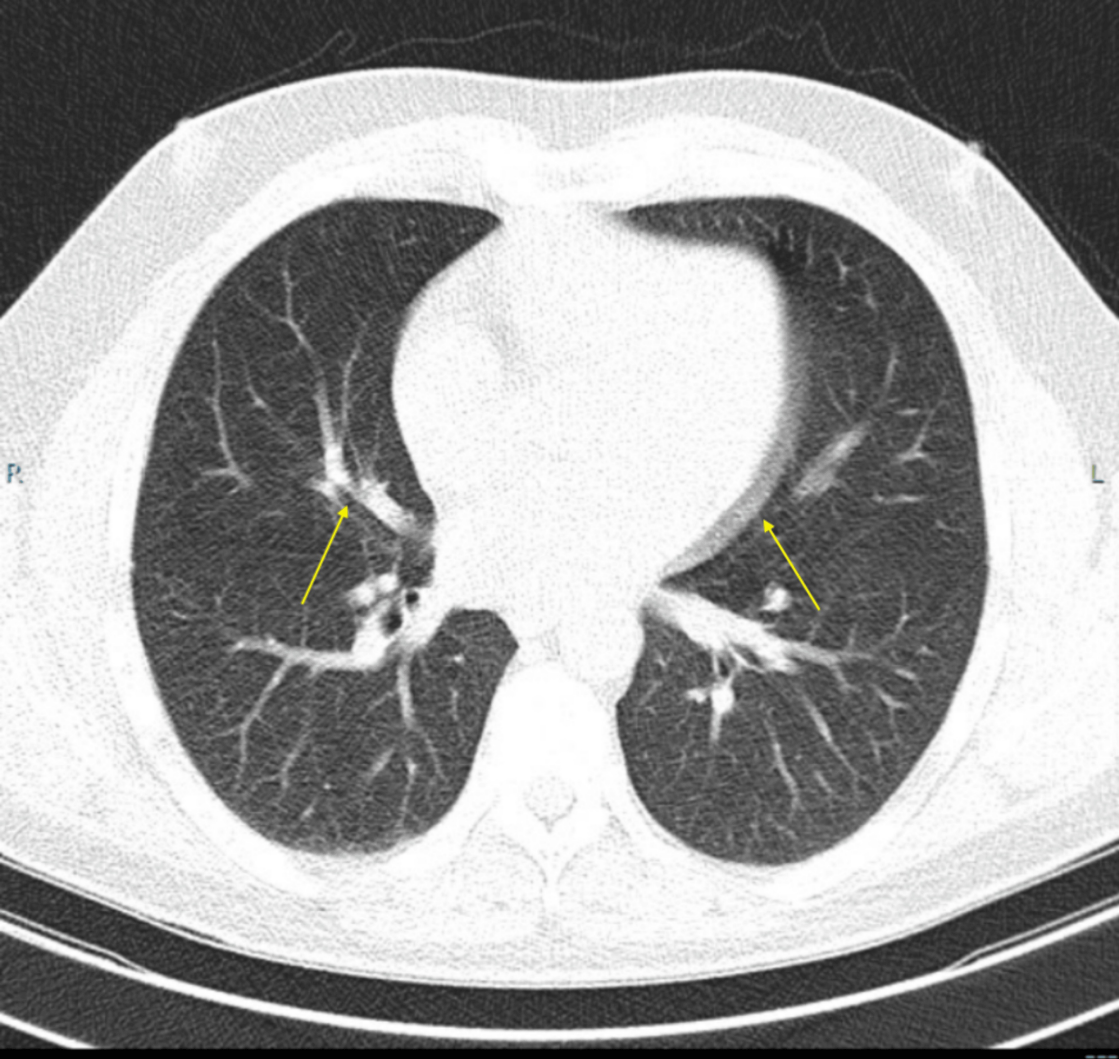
Low-dose CT demonstrating epicardial and coronary artery calcifications The yellow arrow on the right in points to the epicardium, which showed calcifications, and the one on the left points to coronary atherosclerosis.
